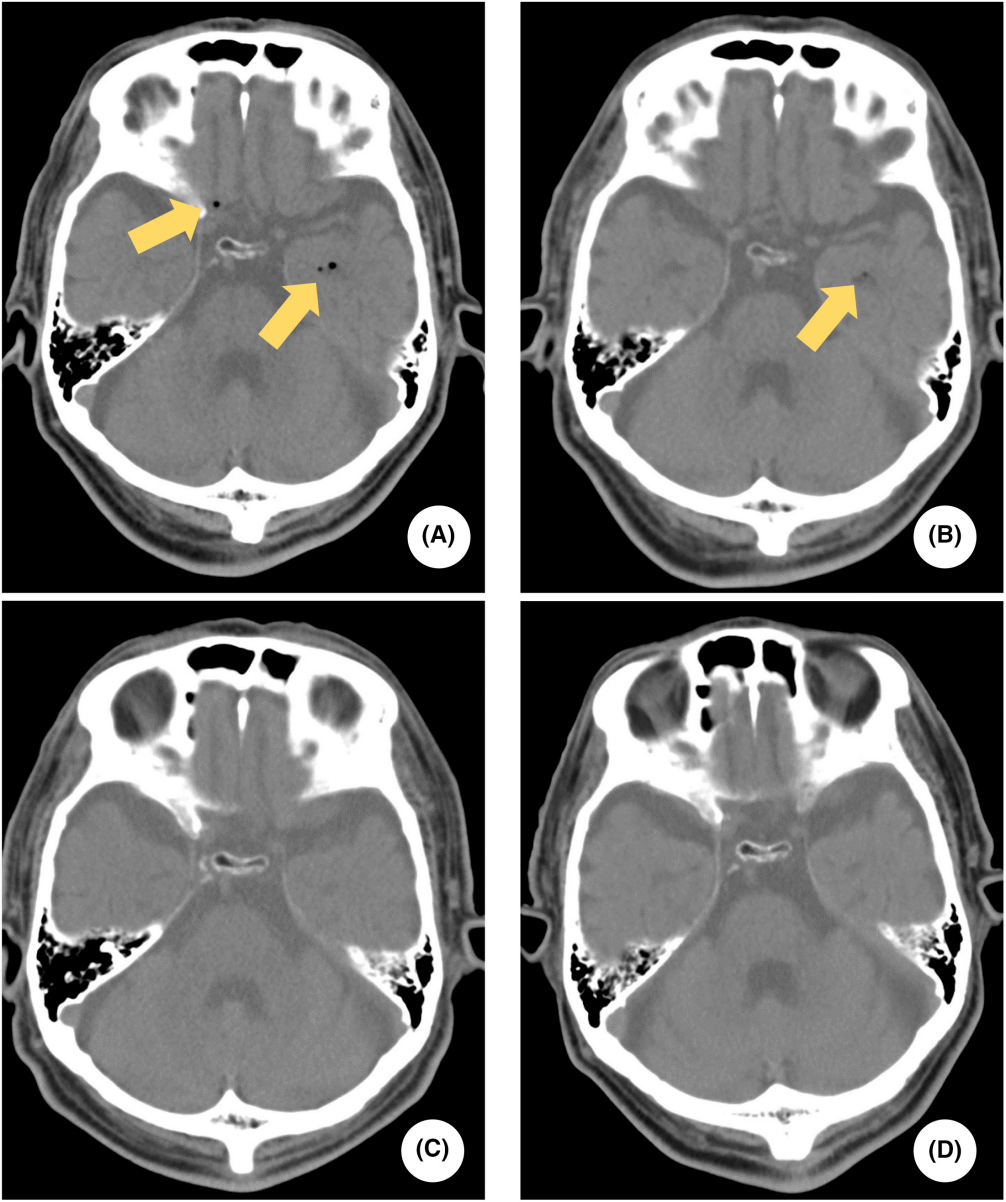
Head computed tomography (CT) of the patient. Pneumocephalus (yellow arrows) is shown in A and B of the head CT image. A：Day 1, B：Day 2, C：Day 4, D：Day 9.
